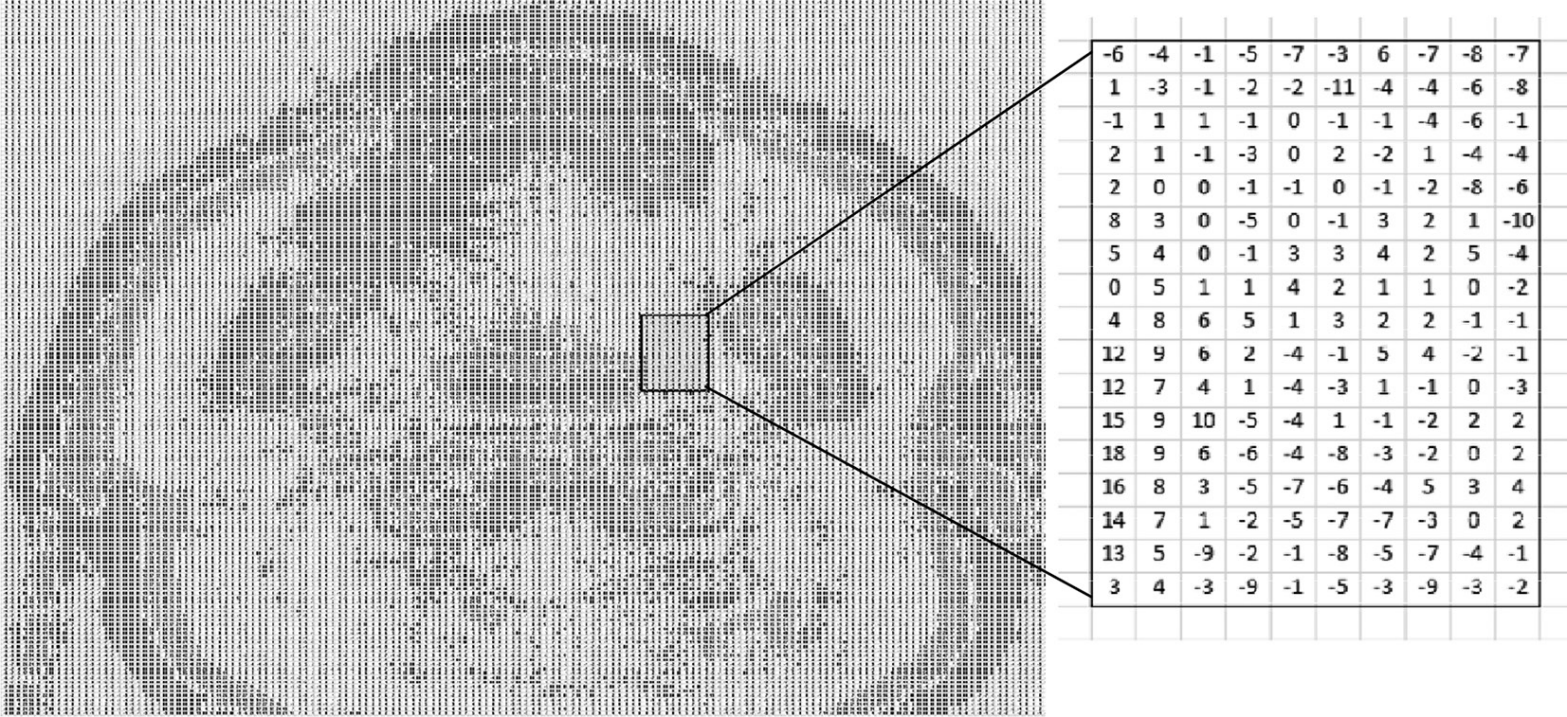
Example of the output of the Matlab code to subtract two images, showing CT number change per pixel
